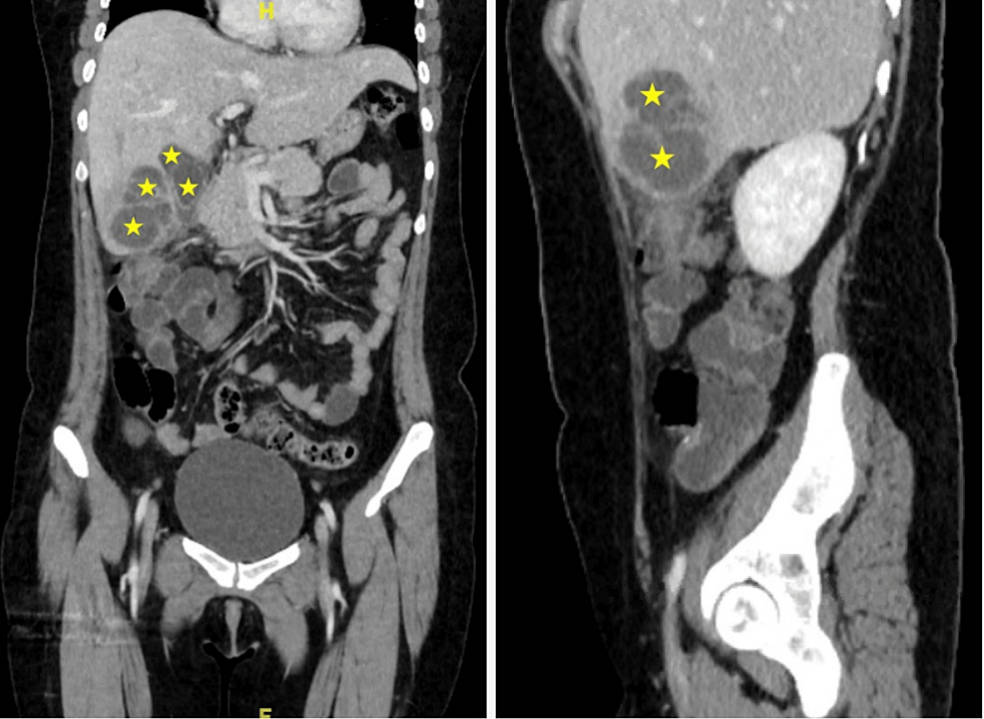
Coronal (left) and saggital (right) views of Computed tomography scan demonstrating liver abscesses (stars)
